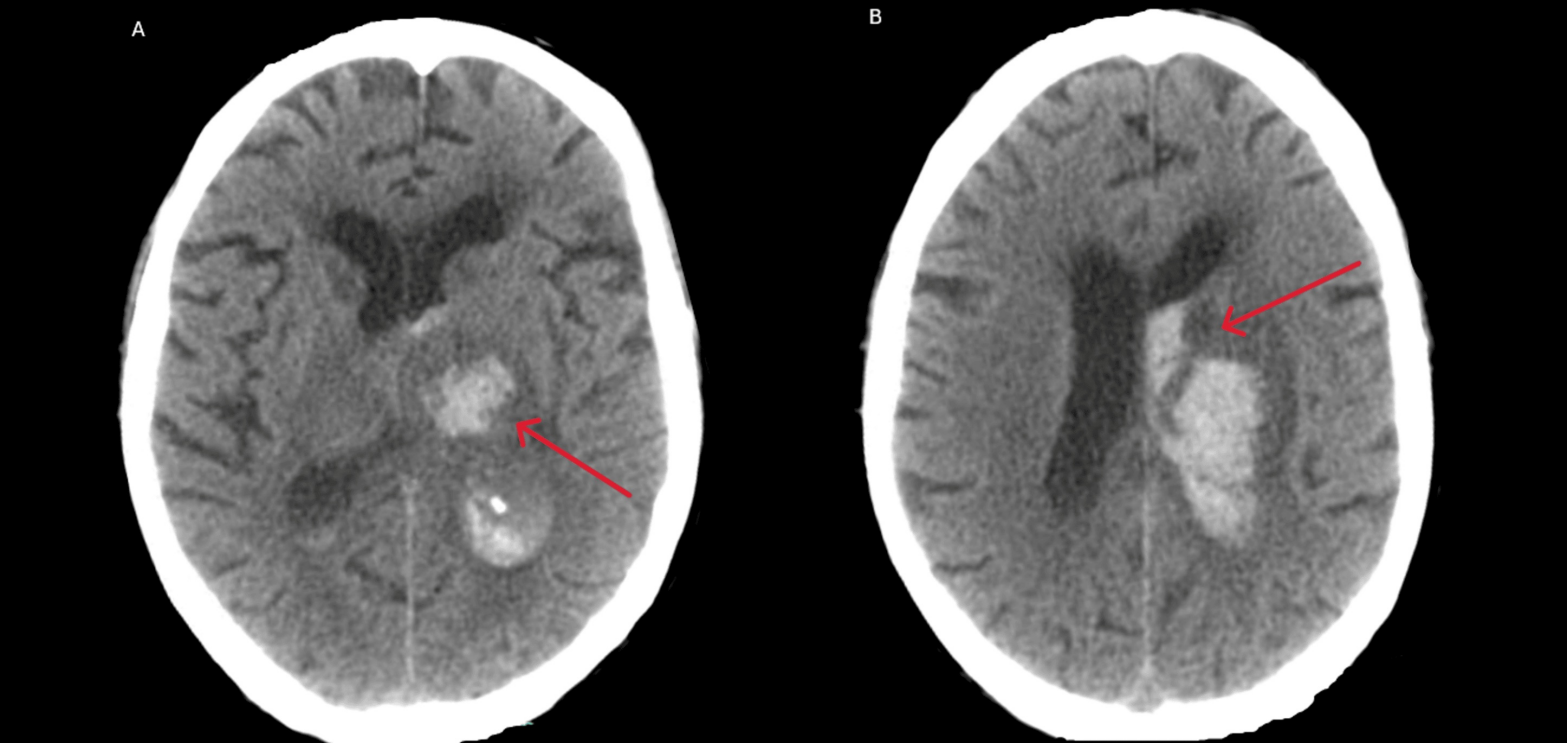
Axial view of the cerebral CT scan showing (A) hyperdensity in the corpus striatum, internal capsule, thalamus, and intraventricular area compatible with hemorrhage and (B) hemorrhagic lesion with signs of hydrocephalus CT: computed tomography
